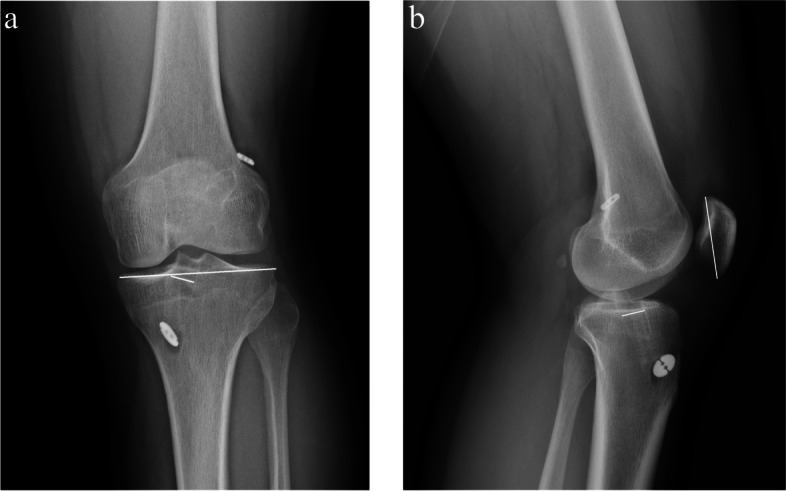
a Measurement of percentage of tunnel widening in anteroposterior view of radiographs. b Measurement of percentage of tunnel widening in lateral view of radiographs
